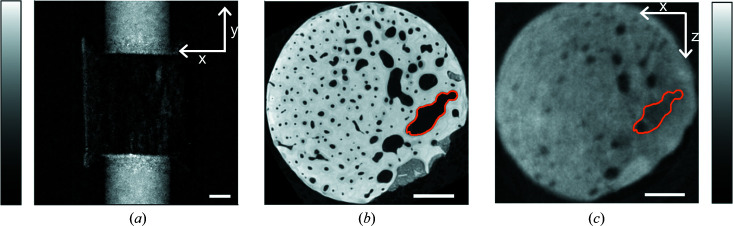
D_2_O uptake in the sample containing more porous bone. (*a*) Two raw neutron detector frames subtracted so that the D_2_O lights up on all sides of the sample. White spots mid-sample are assigned to D_2_O in bigger void spaces, corresponding to the area marked in orange in panels (*b*) and (*c*). (*b*) A typical cross section through the laboratory-based microtomography X-ray data, with a bigger void marked in orange. (*c*) A typical cross section through the phase-retrieved neutron data, with the bigger D_2_O-filled void marked in orange. All scale bars equal 1 mm.
